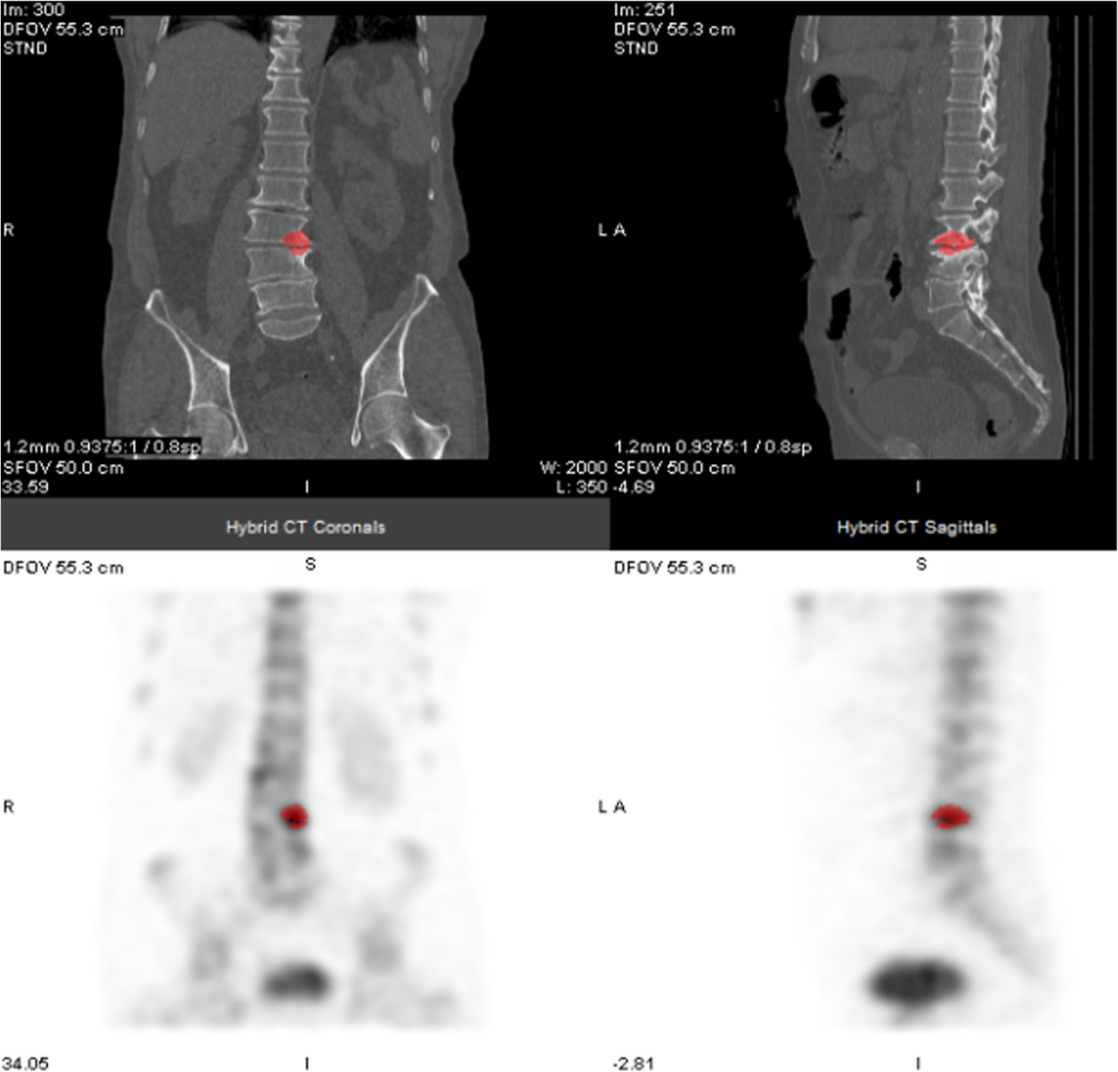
A local case study. Using GE’s Q.Volumetrix MI (GE Healthcare) the SUV_max_ at the confirmed site of fusion in the lumbar spine was found to be 28.7 (SUV_mean_ = 15.4), which sits comfortably above Kuji et al.’s normal range (but also within the range derived for metastases) (Kuji et al. 2017). The SUV_mean_ across all CT-segmented bone in the field of view was 3.48, which was lower than expected according to Kaneta et al.’s normal SUV_mean_ range (4.4 ± 0.5). Automated thresholding was employed to delineate the site. Is any of this more useful than visual interpretation? The quantitative result at the fusion site might have supplemented visual interpretation with something of prognostic value. In addition, comparisons could have been made upon follow-up SPECT-CT, enabling better ongoing characterisation of pain generation. Clearly, however, precision is still lacking
